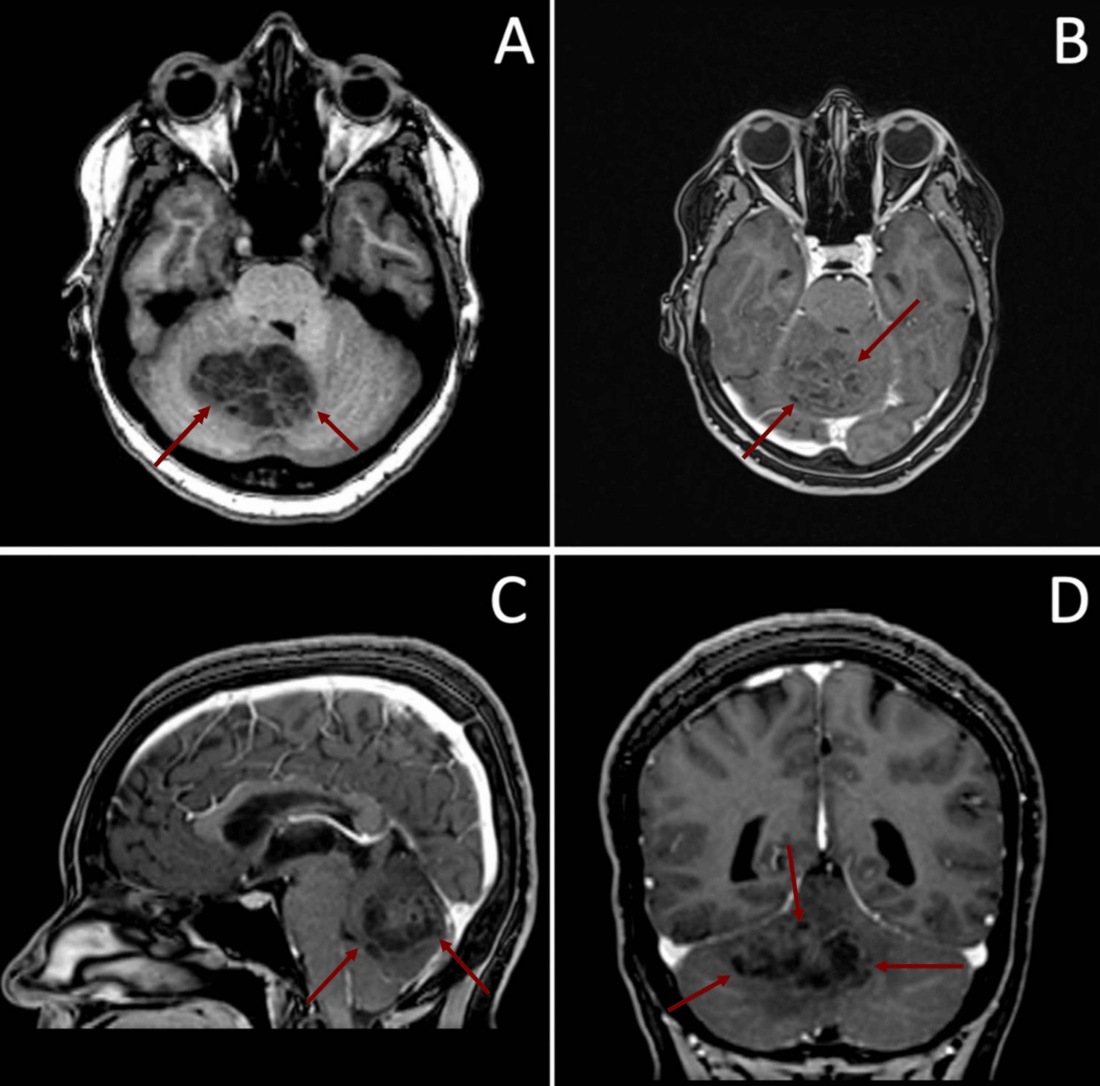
(A) Axial T1-weighted MRI showing heterogeneous space-occupying lesion on posterior fossa (red arrows); (B) axial post-gadolinium T1-weighted MRI showing slight post-contrast reinforcement (red arrows); (C) sagittal contrast-enhanced MRI showing slight post-contrast reinforcement (red arrows); (D) coronal contrast-enhanced MRI showing heterogeneous space-occupying lesion on posterior fossa (red arrows). MRI: magnetic resonance imaging
